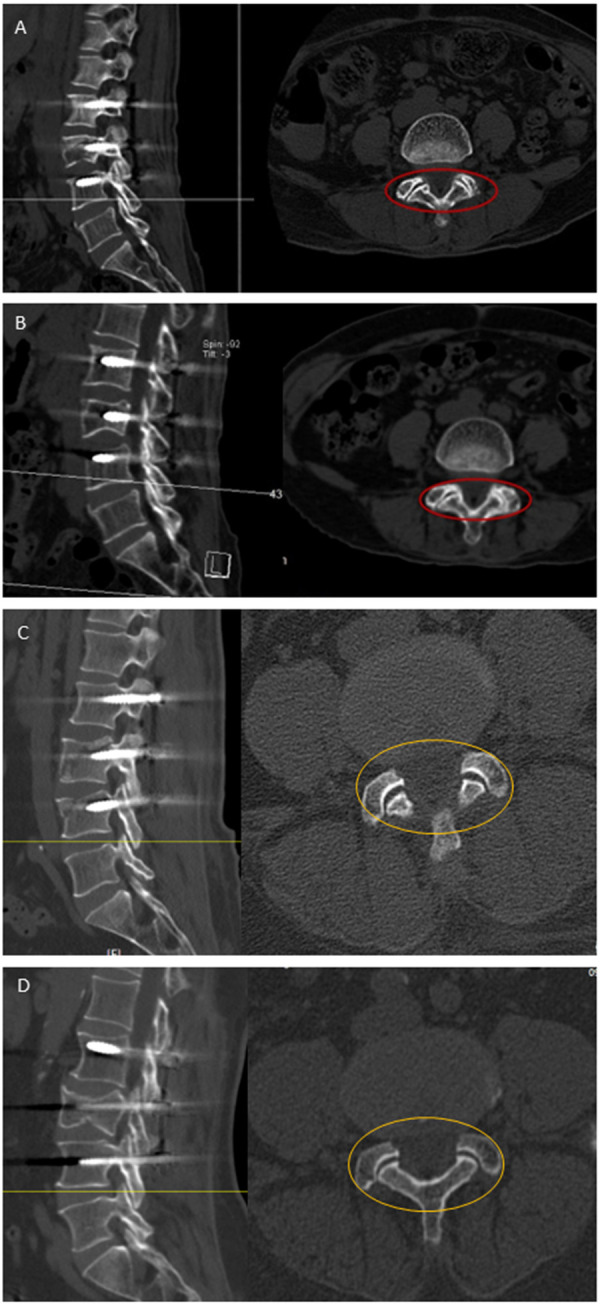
Radiographic change of bilateral facet joint degeneration in adjacent segments after short segment fixation. (A,B) Case 1, female, 61 years old, L3 fracture. A CT scan executed 1 week post-op (A) and 1 year post-op (B) clearly shows bilateral facet joint degeneration in adjacent segments. The red circle indicates a marked reduction or potential fusion of the joint space in the postoperative facet joint. (C,D) Case 2, male, 61 years old, L3 and L4 fracture. A CT scan executed 1 week post-op (C) and 17 months post-op (D) clearly shows bilateral facet joint degeneration in adjacent segments. The yellow circle indicates a marked reduction in joint space and the presence of bone hyperplasia in the postoperative facet joint.
